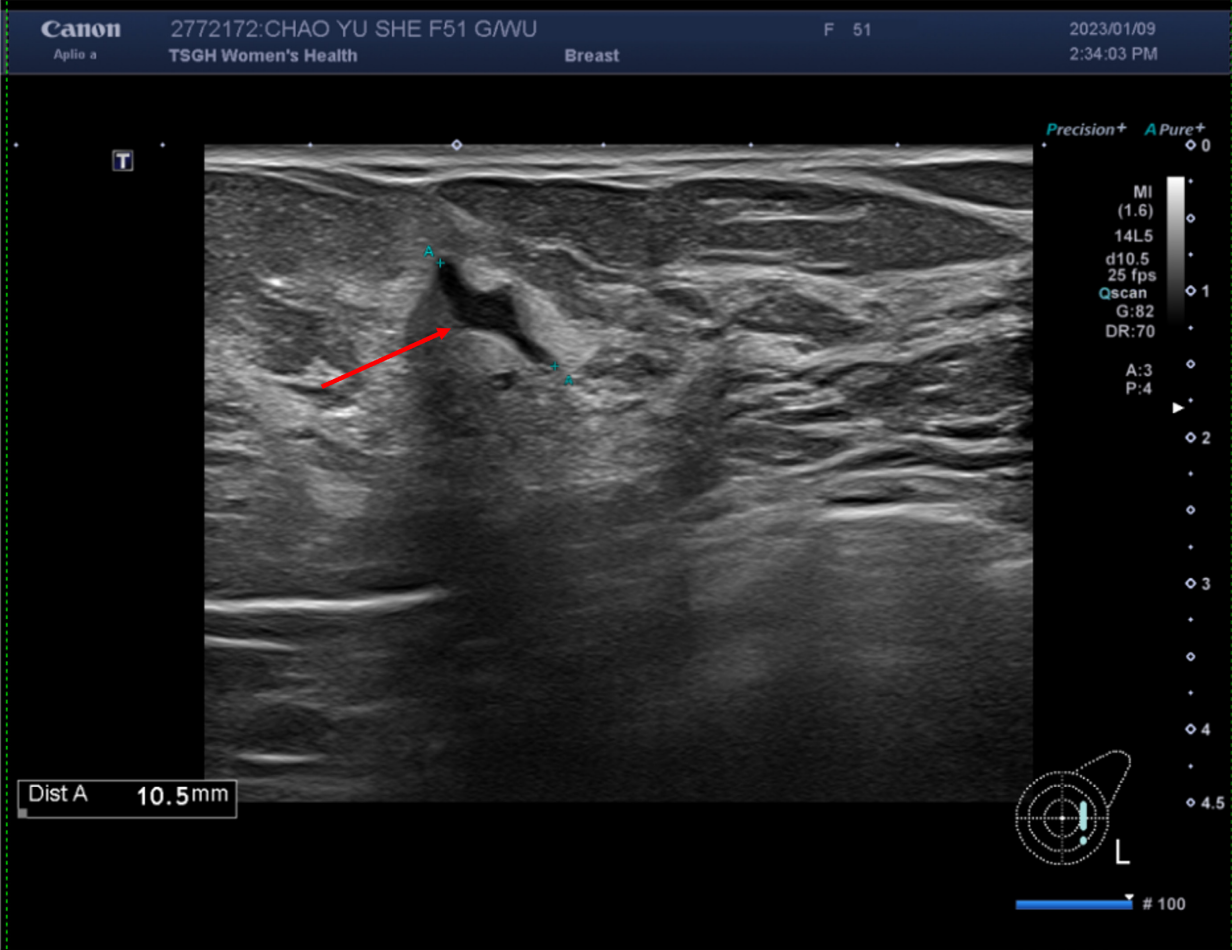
Sonography of the breast. A 10.5 mm lobular mass with poorly defined margins at the 3 o'clock position of the left breast (red arrow).
